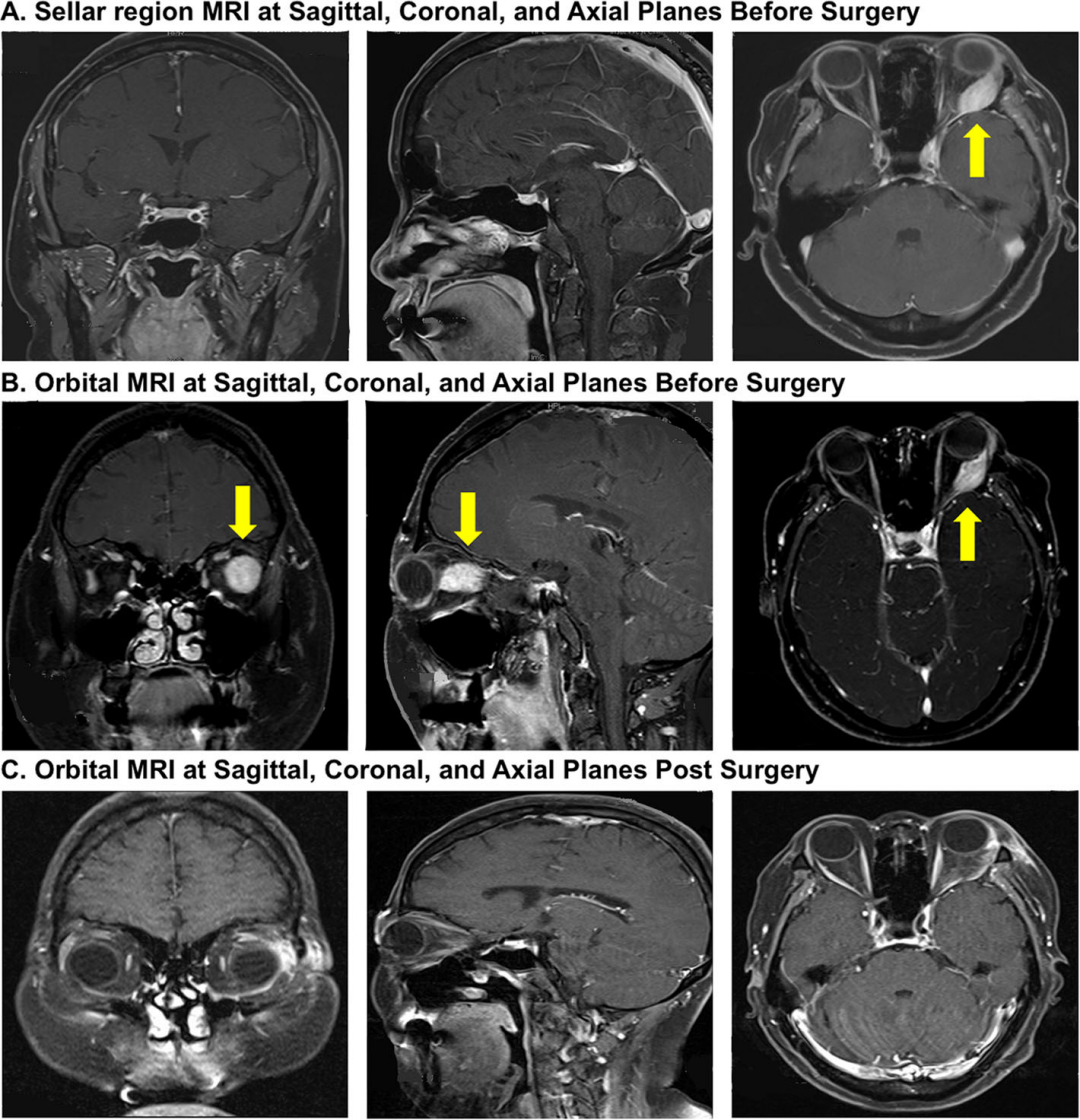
The image profiles of the EAS patient. a Pre - operational sellar region MRI shows normal pituitary but orbital mass around lateral rectus muscle of the patient’s left eye. b Pre - operational orbital MRI displays a tumorous lesion around lateral rectus muscle of the patient’s left eye. c Post - operational orbital MRI shows normal lateral rectus muscle of eye
